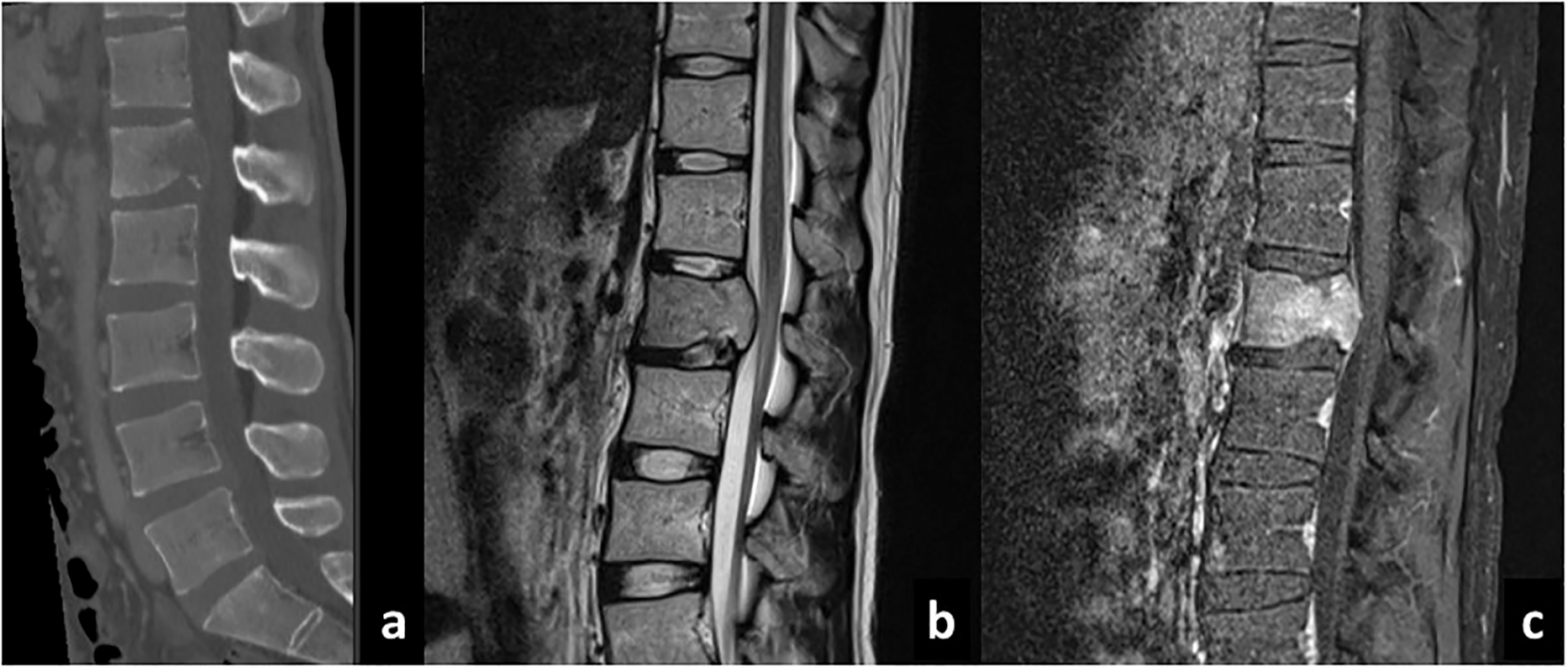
Sagittal CT (A), T2-weighted (B), and gadolinium-enhanced T1-weighted (C) MRI sequences of the spine showing development of metastatic lesion.
